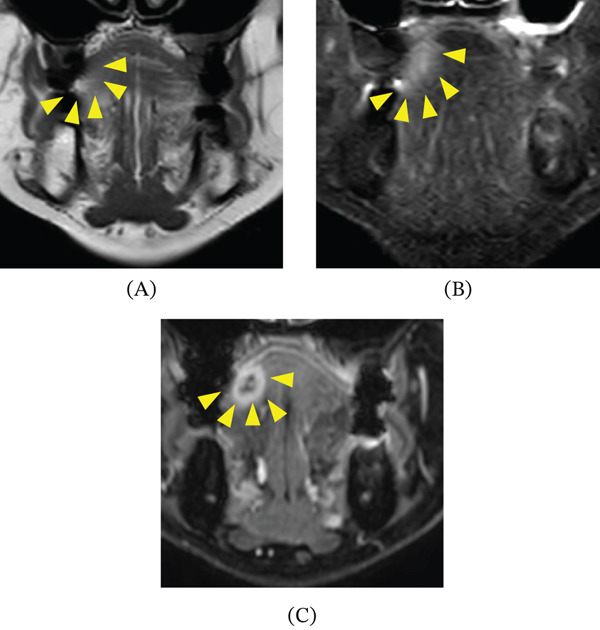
MRI findings. (A) T1‐weighted image showing low signal intensity. (B) STIR image showing faint high signal intensity. (C) Contrast‐enhanced T1‐weighted image showing peripheral enhancement with relatively low central enhancement.
